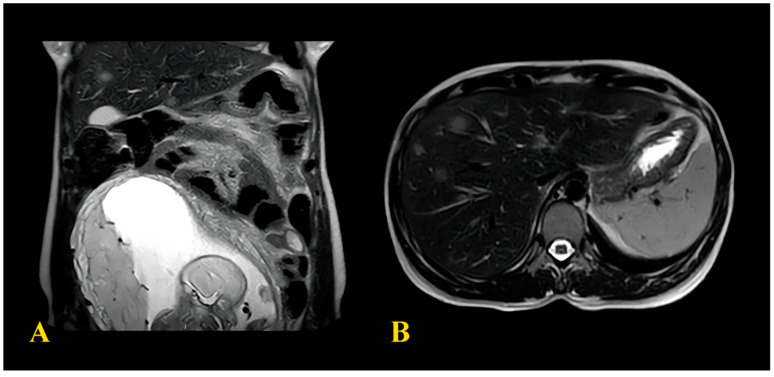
(A) MRI images showing the formation in amniotic sac; (B) CT image showing the hepatic metastases.
